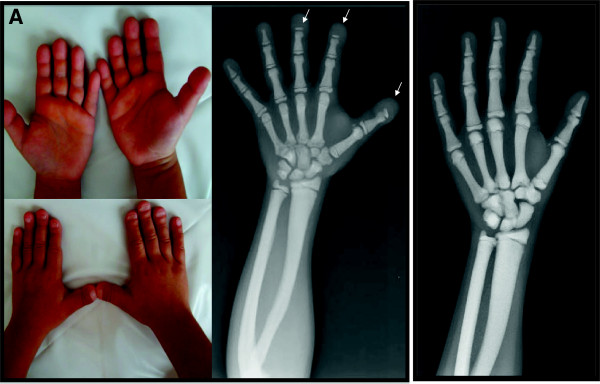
Panel A: Typical finding of acro-osteolytic distal phalanges on X-rays and wrinkled skin over the dorsa of distal fingers and flattened and grooved nails in pycnodysostosis detected in patient 4. Panel B: Osteosclerosis without acroosteolysis detected on radiograph of the patient #8.
